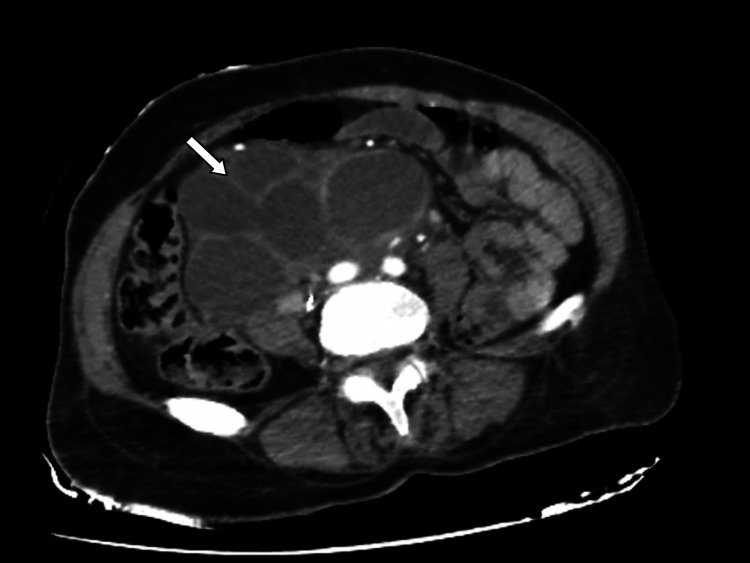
Contrast-enhanced CT scan of the abdomen and the pelvis (axial view) Axial contrast-enhanced computed tomography of the abdomen and the pelvis showing a giant ectopic right kidney (17.7 cm × 13.5 cm), dilated renal pelvis and calyces with multiple central hypodense areas, round in shape, enclosed by an enhancing rim of contrast and peripheral fat stranding (white arrow).
